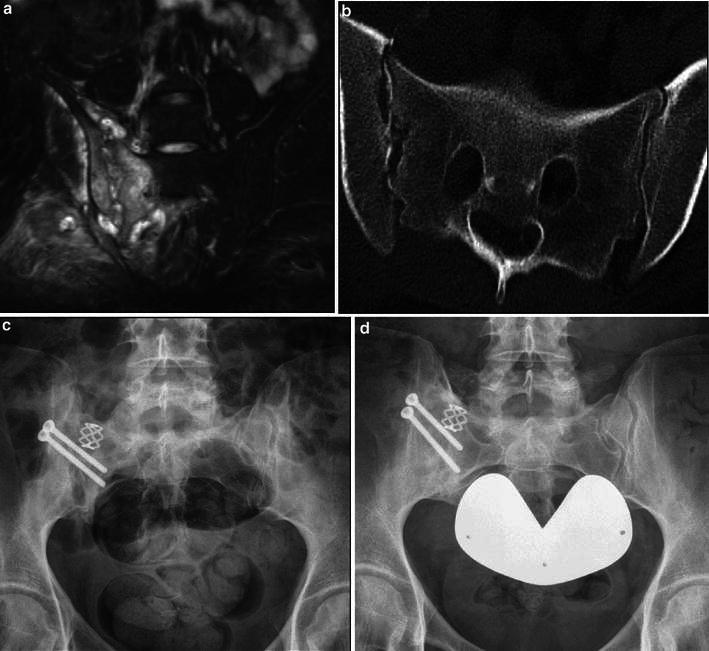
a Case 9: MRI performed after admission showed high signal intensity in the right SIJ and adjacent muscles with abscess formation and bone oedema. b CT revealed widening of the joint space, cavitations and sequestrum formation. c Postoperative radiograph revealed good position of the cage and screws. The patient was allowed to bear weight with assistance after 6 weeks and to fully bear weight after 4 months, after confirmation of bony fusion of the joint. After 1 year, the patient had no complaints and was satisfied. d FU radiographs showed complete bony fusion of the joint. At the last FU visit (49 months postoperatively), she had excellent functional outcome, no pain and no limitations of daily activity. She returned to work and practised sport regularly
